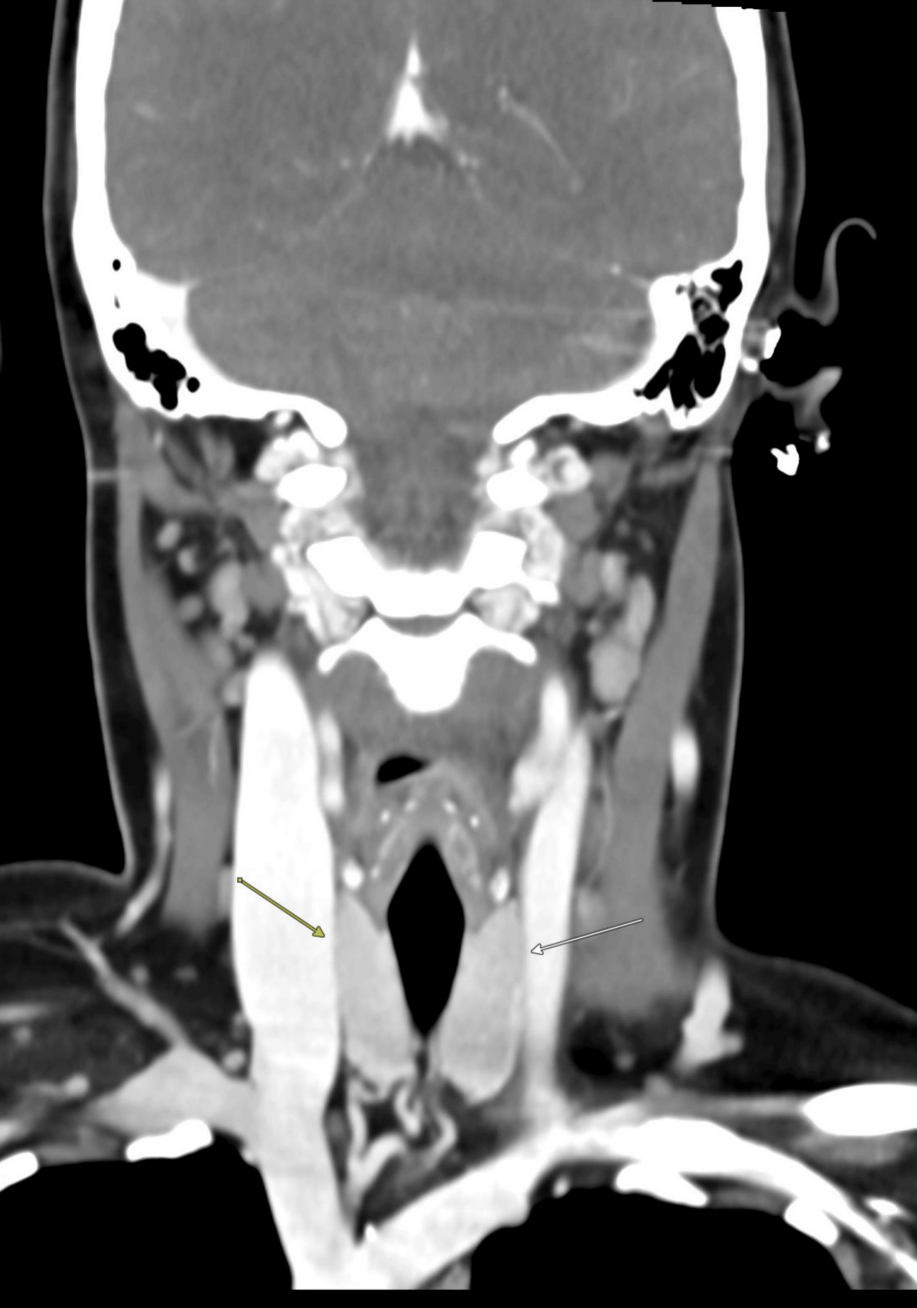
Coronal computed tomography image demonstrating bilaterally homogeneous thyroid lobes (arrows)
